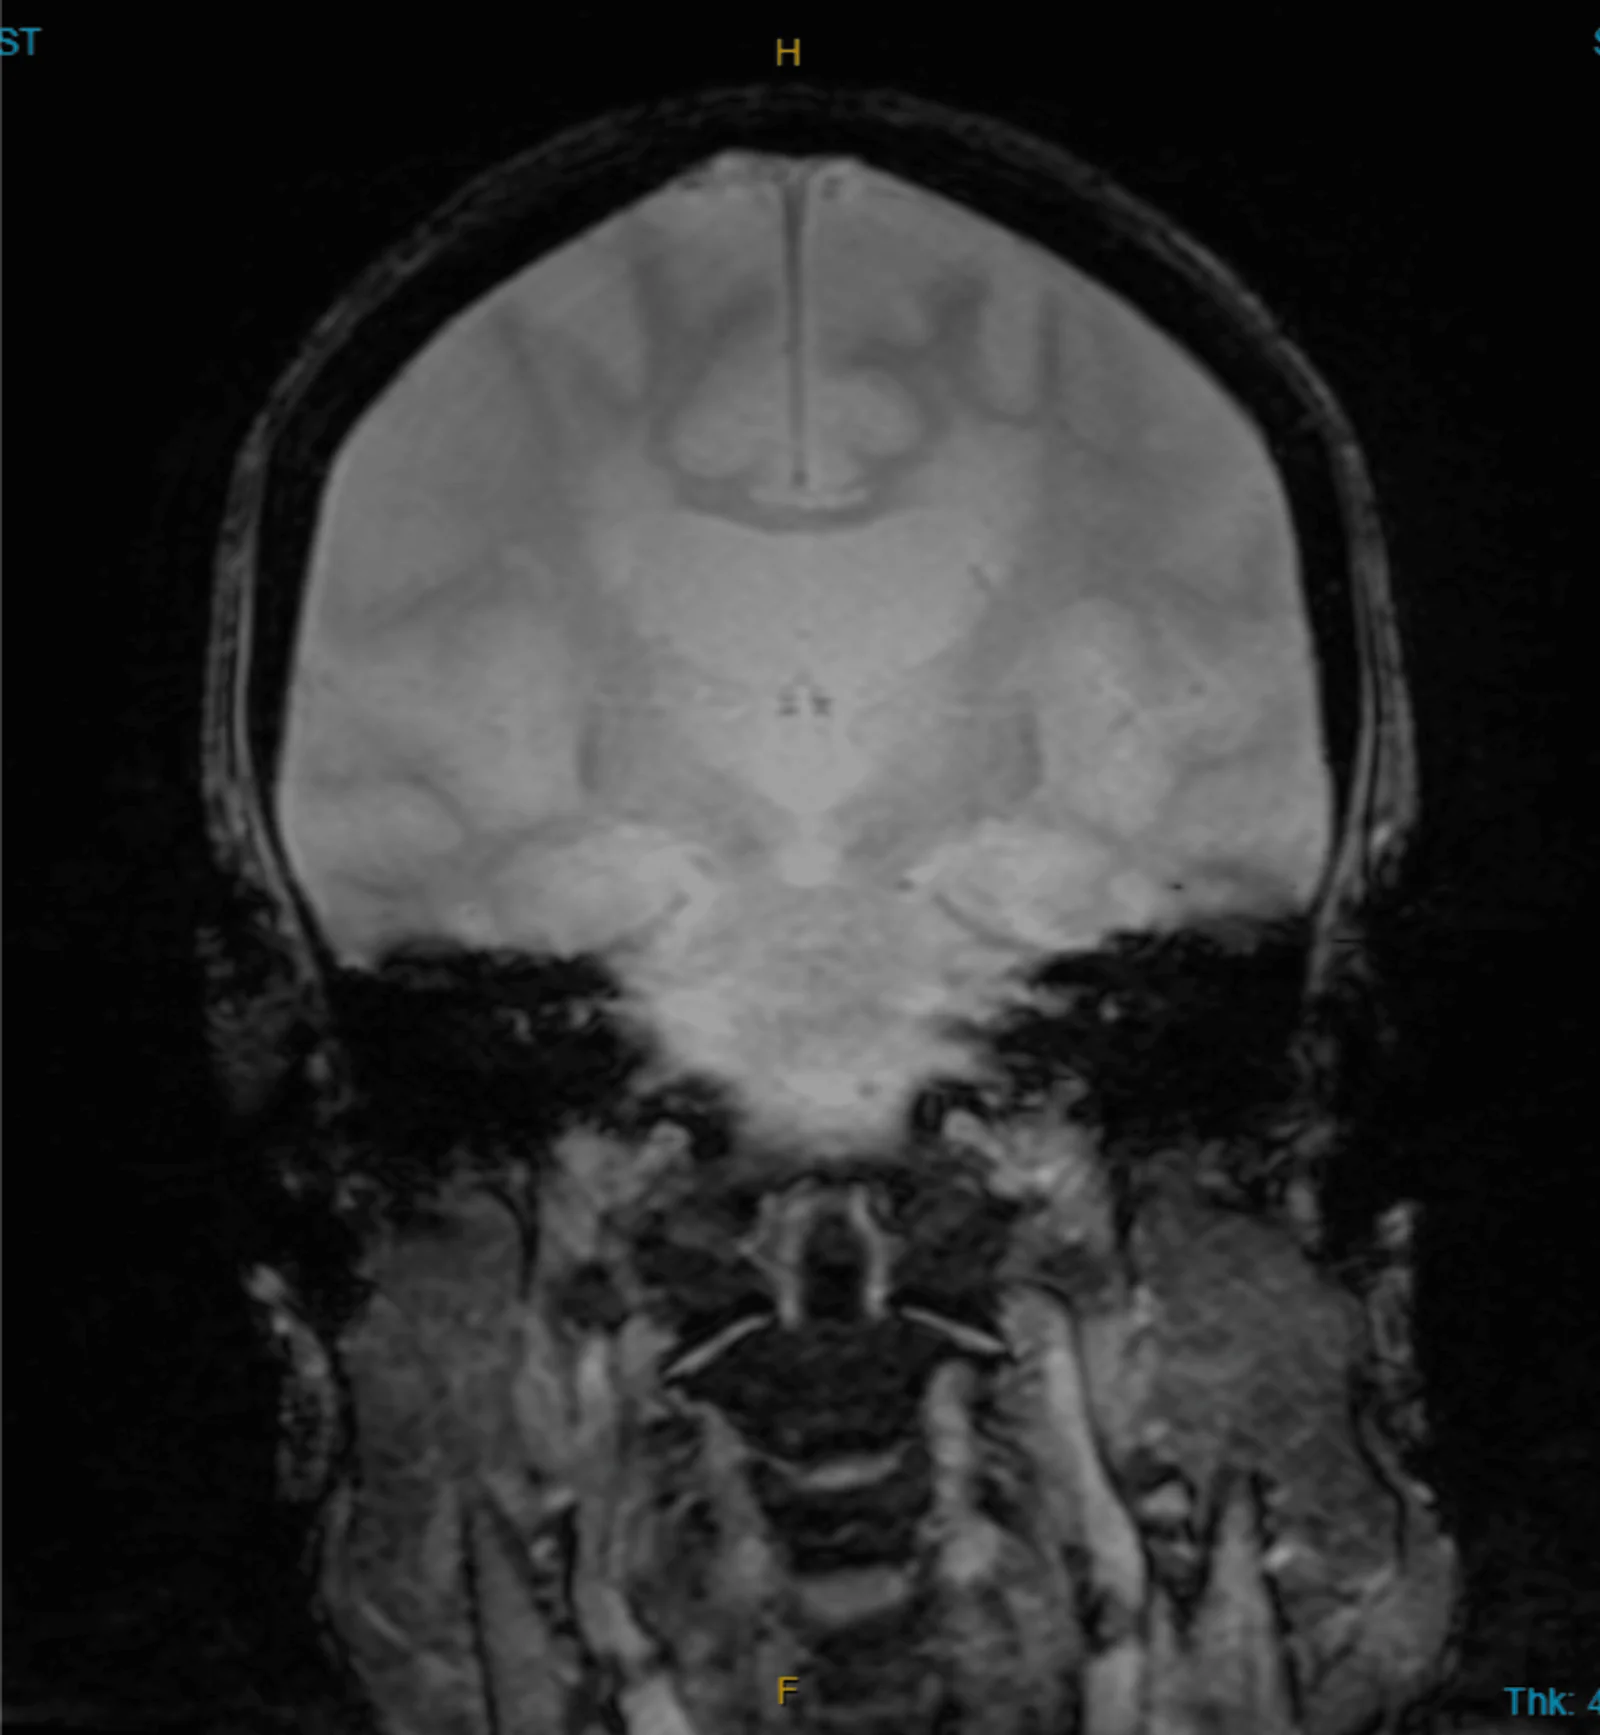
MRI brain: coronal T2 GRE MRI demonstrating susceptibility-sensitive assessment for hemorrhagic abnormalities. No imaging evidence of amyloid-related hemorrhagic co-pathology was identified, including cerebral microhemorrhages or cortical superficial siderosis.
